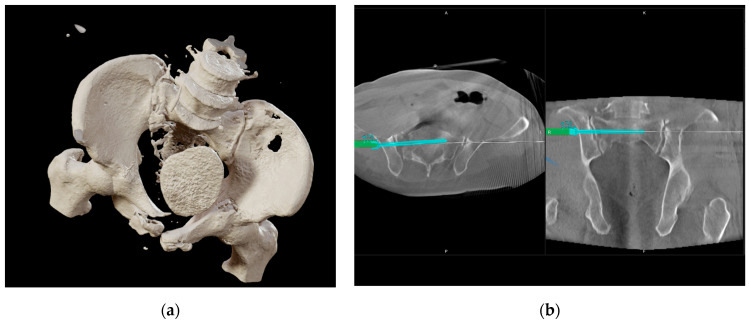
After initial and secondary assessment according to ATLS principles, initial CT diagnostics and primary surgical treatment were performed. (a) Initial 3D CT reconstruction of the pelvis, showing the posterior and anterior fracture dislocation in relation to the bladder; (b) 3D navigation planning of posterior pelvic ring reconstruction for initial stabilization.
